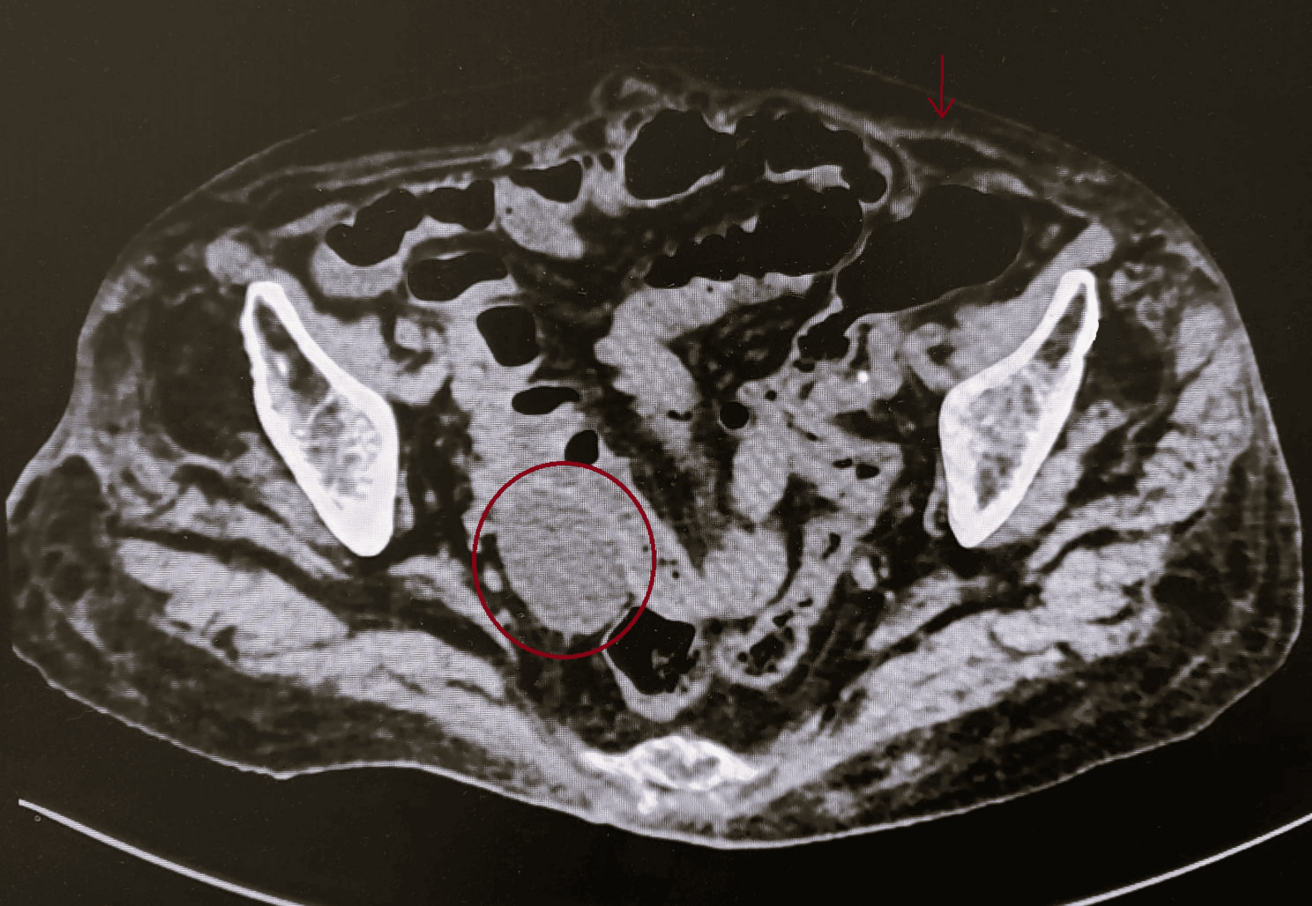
CT axial projection showing an abscess contained adjacent to a jejunal loop (red circle) and pneumoperitoneum (red arrow) CT: computed tomography
